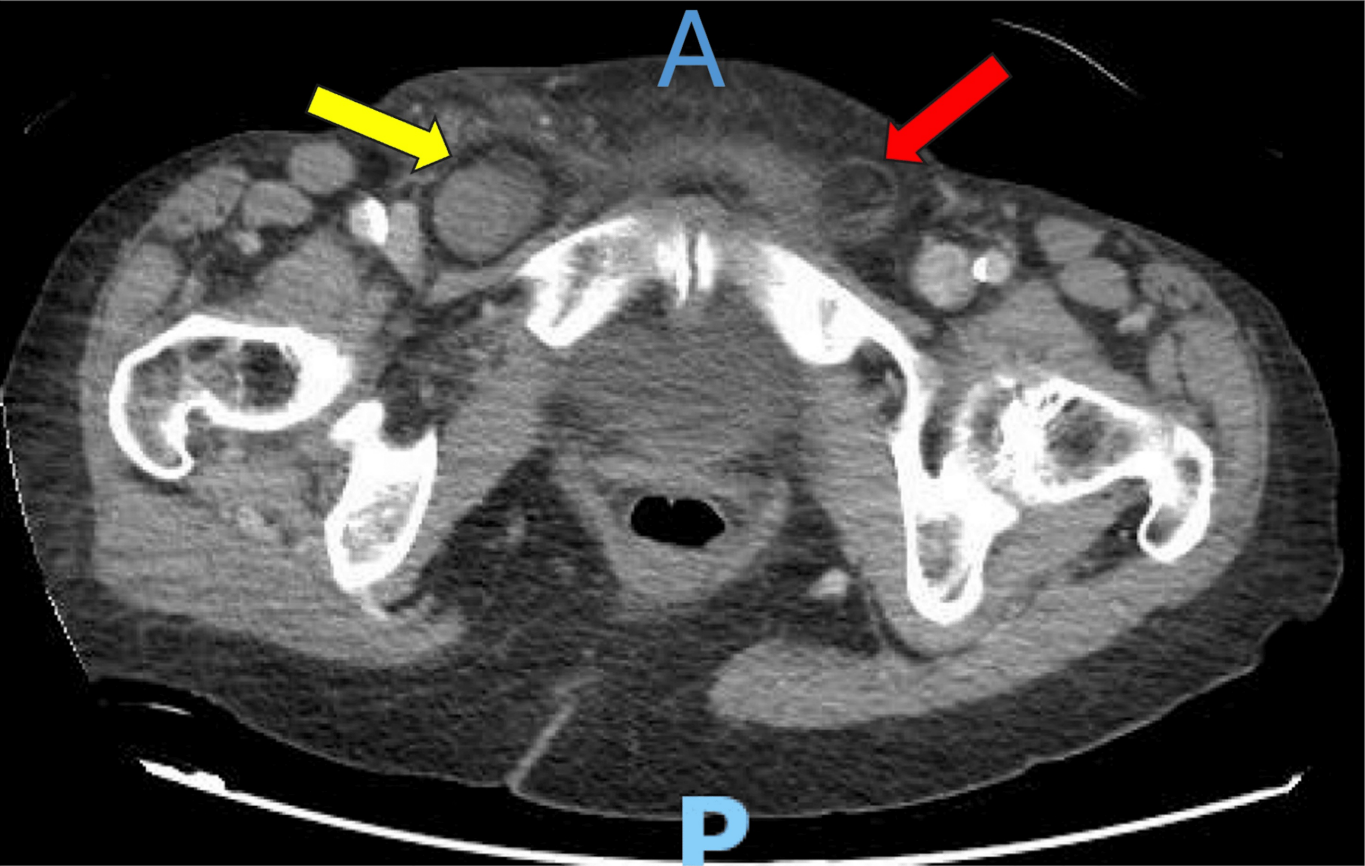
Axial view of the CT of the pelvis with intravenous contrast A is the anterior side; P is the posterior side. The yellow arrow is pointing at the bowel-containing right-sided inguinal hernia. The red arrow is pointing at the fat-containing left-sided inguinal hernia.
